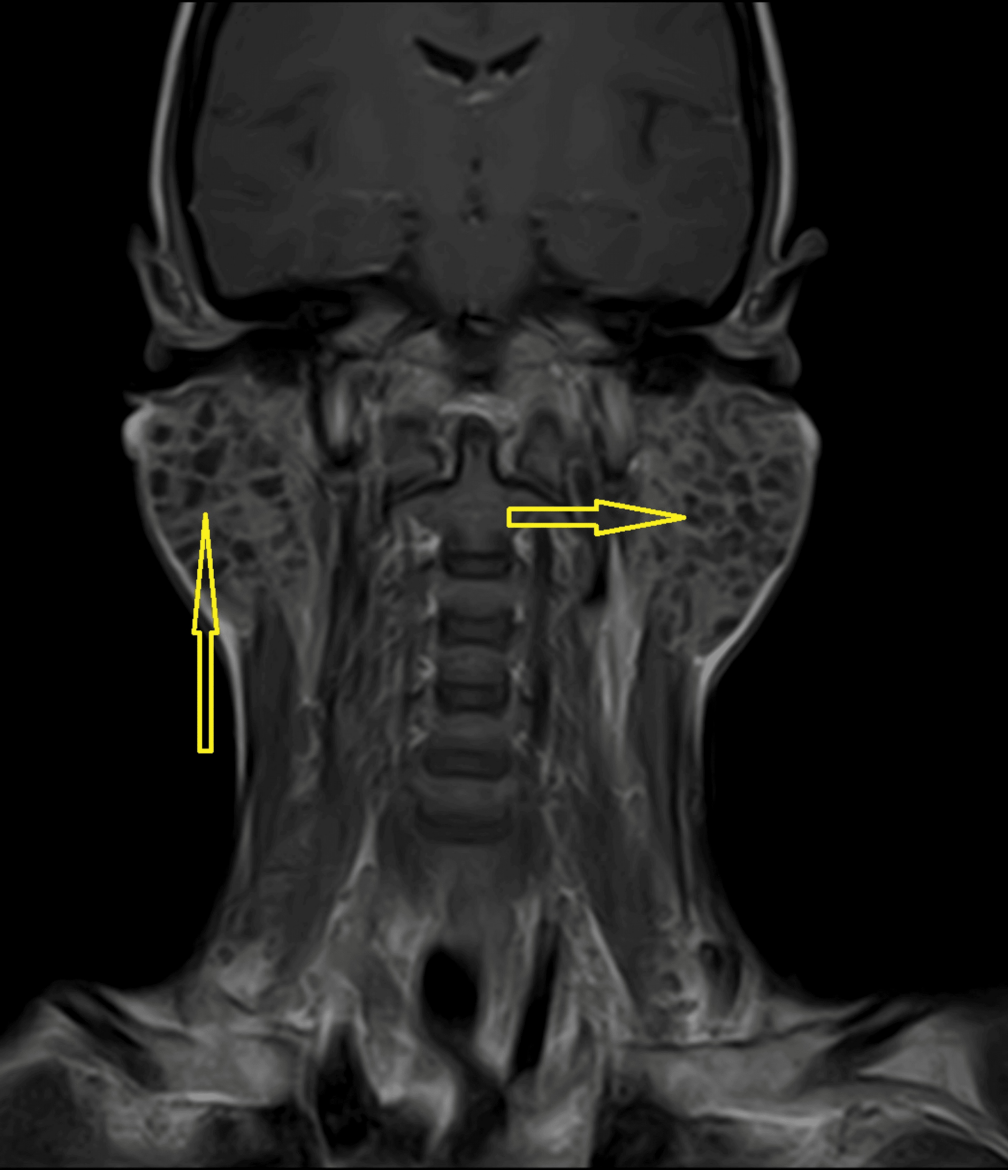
Contrast-enhanced T1-weighted coronal image showing the hypointense lesions in the bilateral enlarged parotid glands
